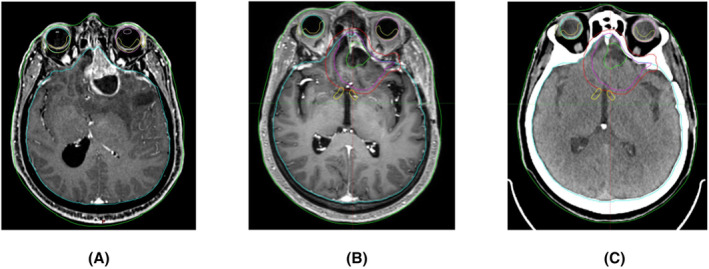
(A) Left frontal lesion radiologically suspected for high‐grade meningioma before surgery. MRI (B) and radiotherapy planning CT (C) after gross total resection: chiasma‐PRV in yellow, GTV in green, CTV in purple, and PTV in red.
